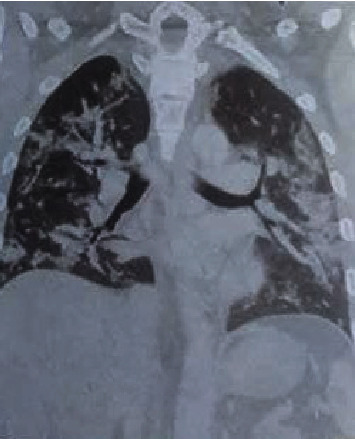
HRCT of chest showing multifocal consolidation with ground glass opacities, interlobular septal thickening, and fibrotic bands in the lungs.
